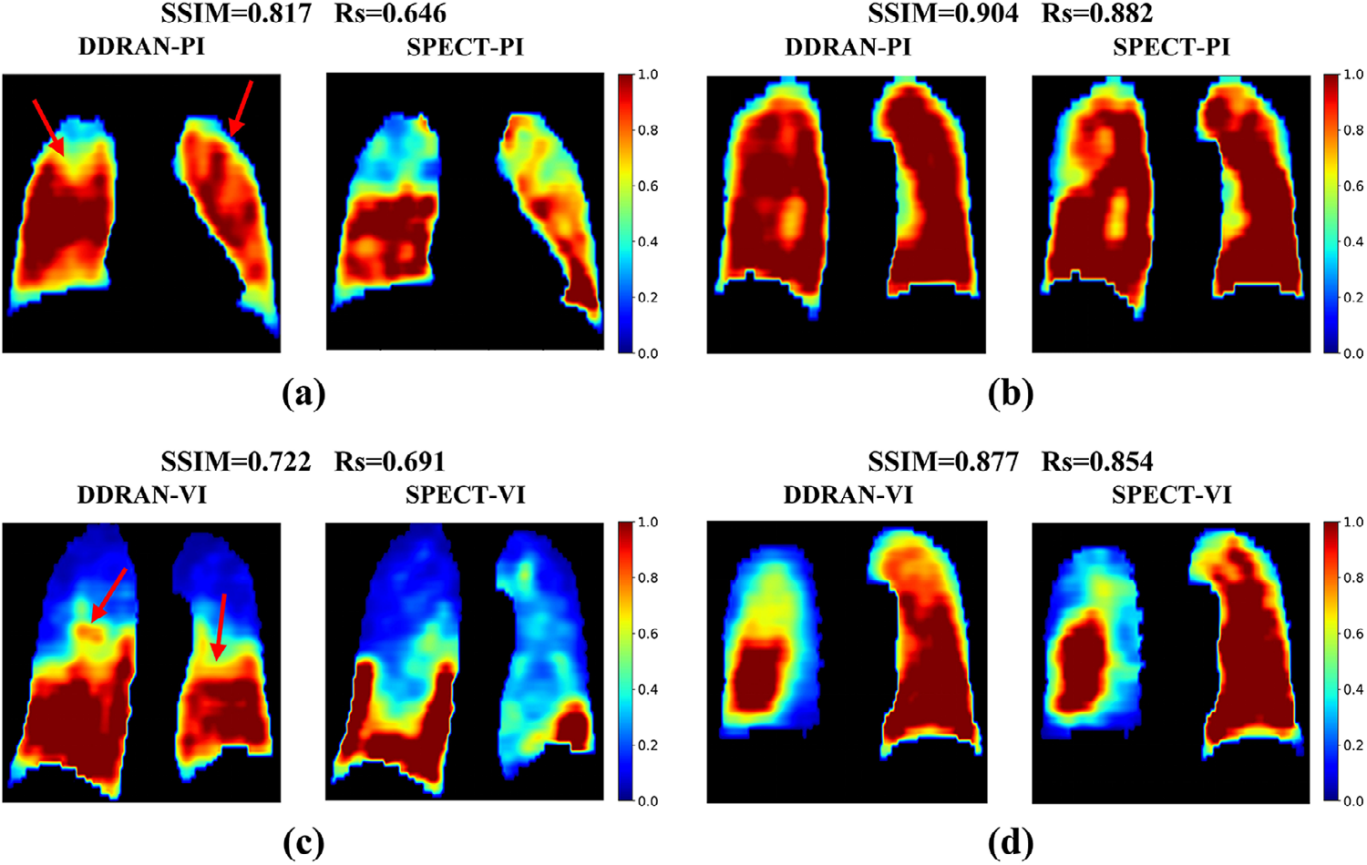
Qualitative results of synthesized lung function images. Four test cases are demonstrated in (a–d) from a view of the coronal plane. The upper row displays the synthesized lung perfusion images, while the bottom row shows the lung ventilation images. For the two well‐performing cases (b) and (d) shown above, our DDRAN model can generate lung functional images that resemble real SPECT images. For the two poorly performing cases (a) and (c), the synthesized lung functional images failed to capture the functional information in some low‐function regions, as indicated by the red arrows in the figures.
